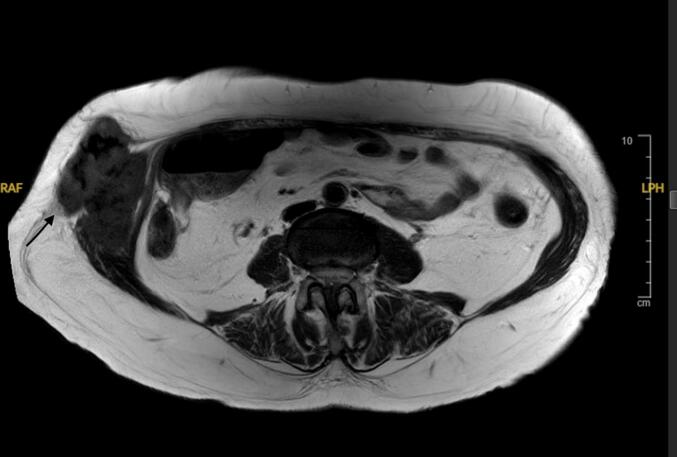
Preoperative T1-weighted MRI revealed a heterogeneous tumor slightly growing in the right rectus abdominis (black arrow).
